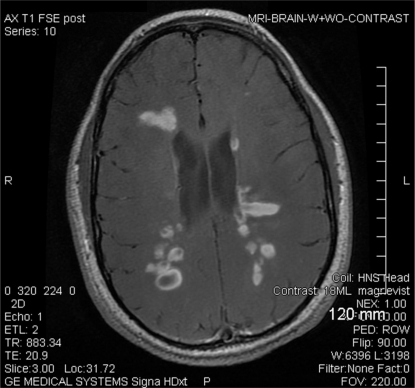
A 25 year old man with a diagnosis of MS had sudden change in neurologic status after discontinuation of natalizumab. He had been on therapy for two years with good response but natalizumab was discontinued due to concerns of increased risk for PML. He switched to glatiramer acetate but after three months from discontinuation of natalizumab, he had acute symptoms of ataxia and fatigue. MRI revealed multiple new T2/FLAIR hyperintensities and T1 enhancing lesions. He was treated with a 5 day course of methylprednisolone and his symptoms promptly resolved (case report and MRI courtesy of Ann Cabot, D.O.).
